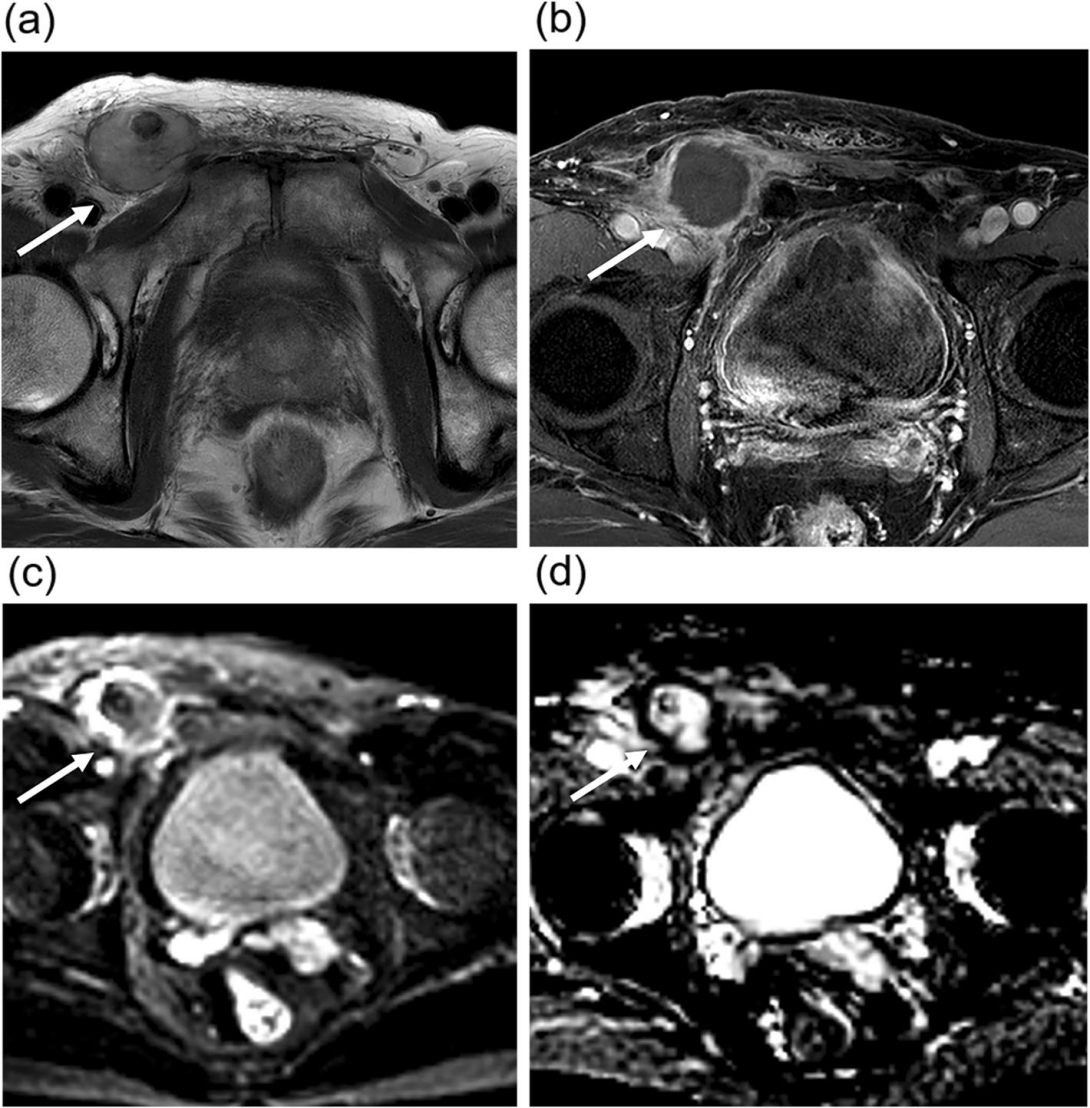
Magnetic resonance images. a A hyperintense mass (arrow) surrounding the hypointense spermatic cord in T2-weighted images. b Peripheral rim enhancement of the mass (arrow) surrounding the spermatic cord in a contrast-enhanced fat suppression T1-weighted image, (c-d) Diffusion restriction along the mass periphery (arrows) of the mass surrounding the spermatic cord on diffusion weighted image (b = 1000) and apparent diffusion coefficient (ADC) map
